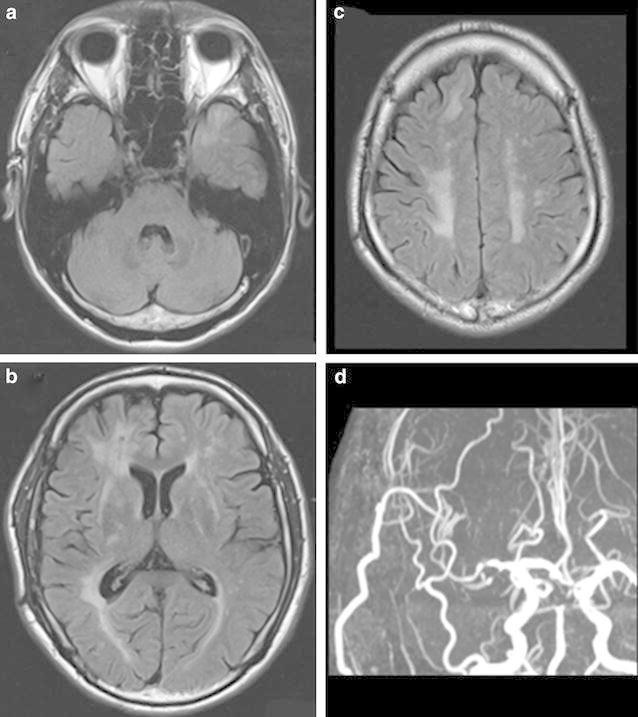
Postoperative MRI shows no remarkable change on abnormal intensity in the anterior temporal lobe (a), external capsules, periventricular regions (b), and white matter (c). MR angiography revealed the patency of the right STA-MCA double anastomoses (d)
